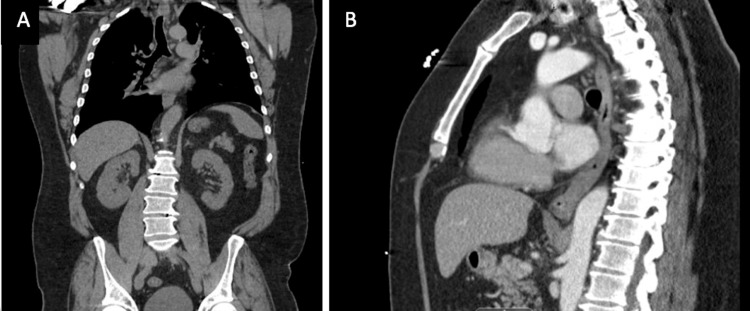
A) CT scan of the thorax abdomen and pelvis without contrast; B) CT scan of the thorax without contrast, depicting clear findings and no evidence of thoracic, abdominal, or osseous metastasis
